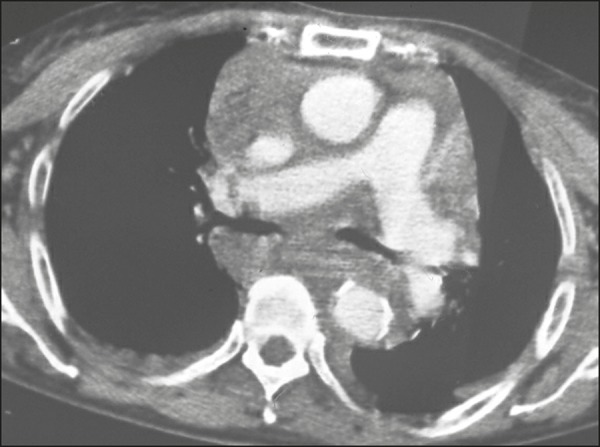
A 21-year-old female gunshot victim. Axial CT scan of the chest after
